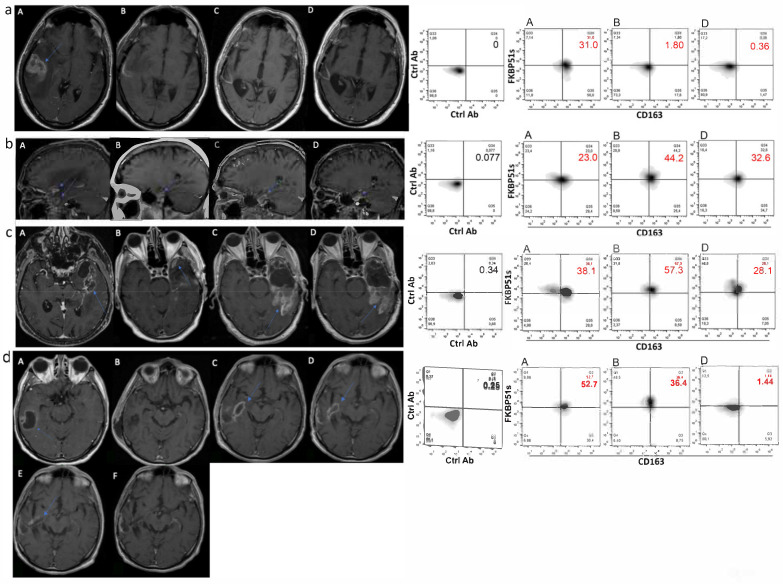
MRI and CD163/FKBP51s+ monocytes on follow-up. Axial T1 images after contrast medium administration: the glioblastoma before surgery in A, the immediate post-operative image in B, post-radio-chemotherapy in C, and post-adjuvant temozolomide (TMZ) in D. On the right, correspondent flow cytometry of CD163/FKBP51s+ monocytes. (a) The glioblastoma before surgery in the right temporal lobe. The immediate post-operative image shows a macroscopically complete exeresis of the tumor. Images C and D show no disease progression. (b) The glioblastoma before surgery in the right temporal lobe. The immediate post-operative image shows a residual tumor. Images C and D show the stability of the residual tumor. (c) The glioblastoma before surgery in the left temporal lobe. The immediate post-operative image shows a residual tumor. Image C highlights a disease progression, which tends to be stable in subsequent D control. (d) The glioblastoma before surgery in the right temporal lobe. The post-operative image shows a macroscopically complete exeresis of the tumor. C and D images show a thick impregnation area along the deepest portion of the surgical cave suspected for disease progression, which tended to regress in subsequent controls (E, F).
